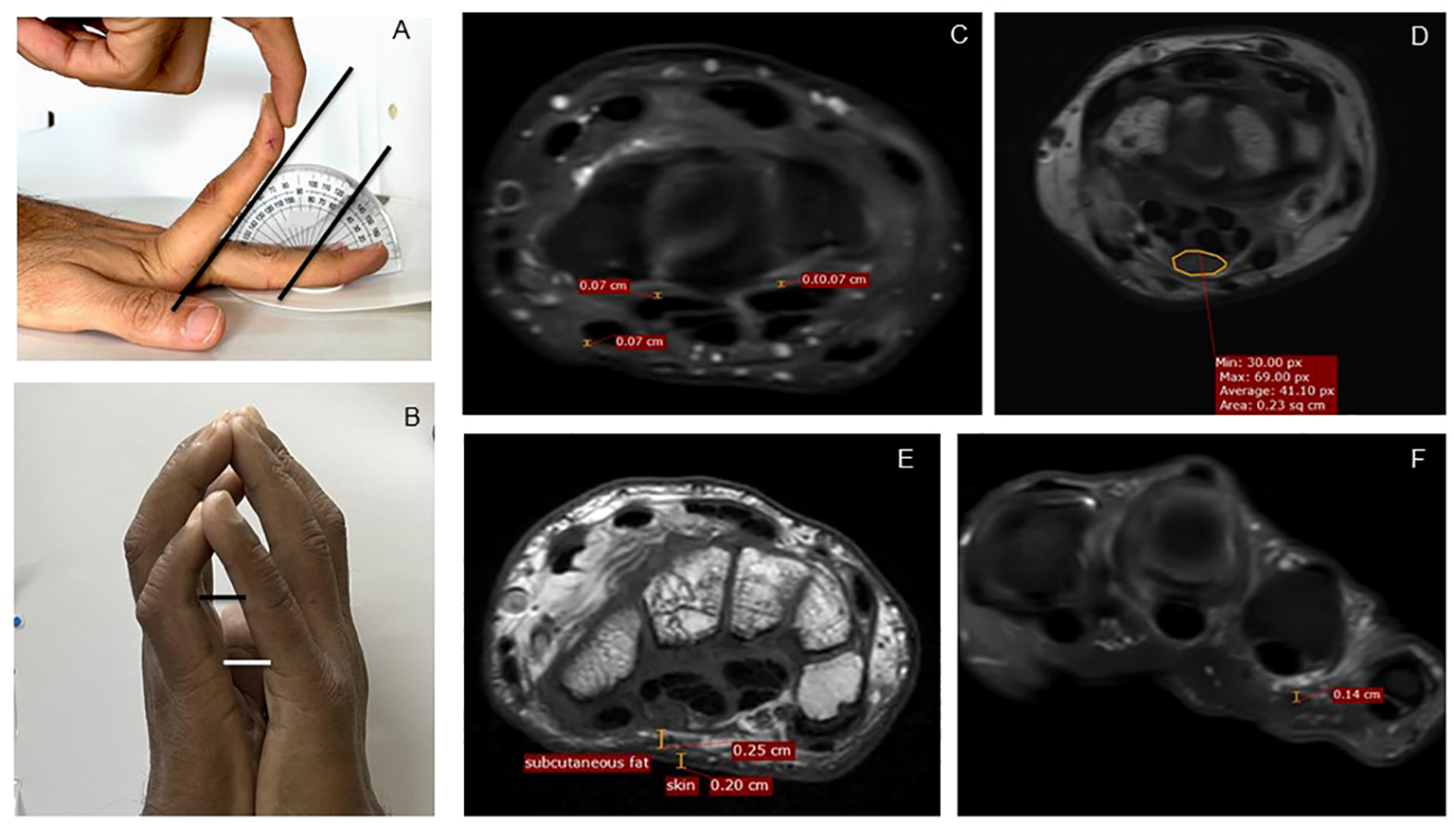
Measurement methodology, clinical and MRI. (A) Measurement of maximum passive extension at MCP joint of the left second finger, here showing 50° extension. (B) Measurements of distance between the fifth proximal interphalangeal joints and the fifth MCP joints as a quantification of the prayer sign. (C) Measurement of tenosynovial thickness at the level of carpal tunnel. (D) Measurement of median nerve area at the level of the carpal tunnel. (E) Measurement of skin and subcutaneous fat. (F) Measurement of palmar fascia overlying the fourth flexor tendon on PDFS images. MRI, magnetic resonance imaging; PDFS, proton density fat saturation.
